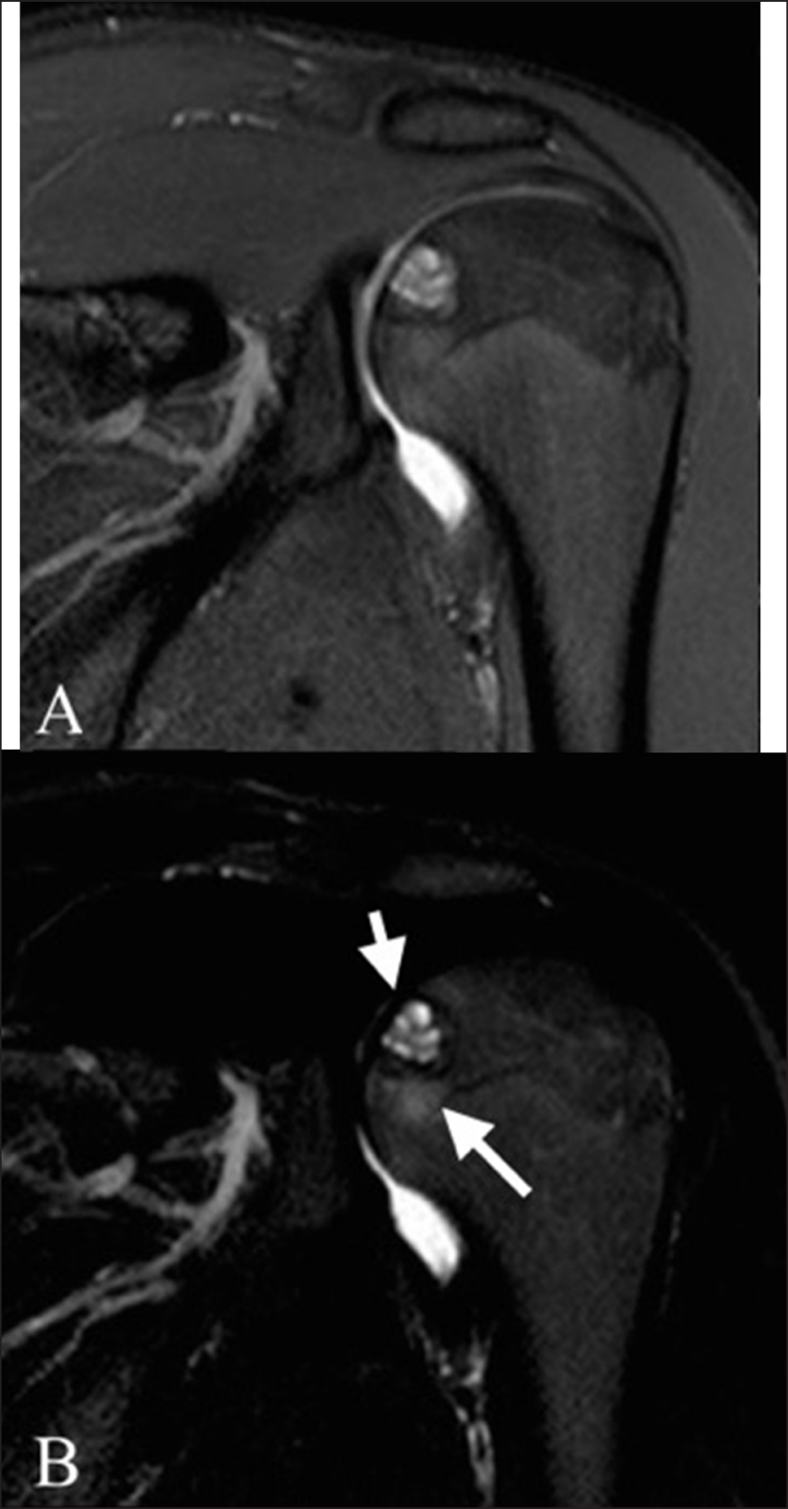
Coronal dual TSE FS images of the left shoulder. (A): proton density weighted image: well described lesion in the proximal humeral epiphysis without disruption of the subchondral bone plate, (B): T2-weighted: central high signal intensity (short arrow), limited bone marrow edema (long arrow).
